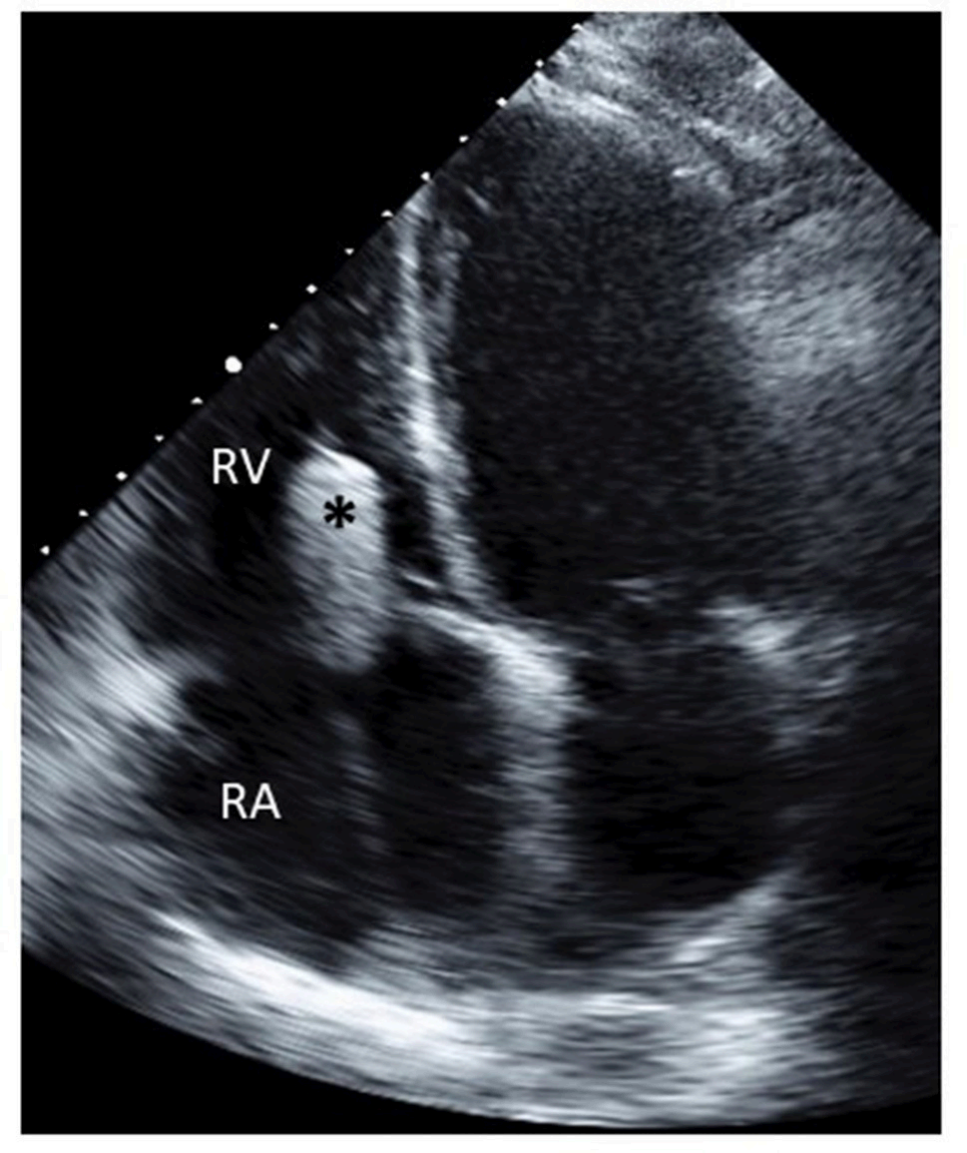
Transthoracic echocardiography showing the FORMA spacer (*) positioned in the tricuspid valve.
